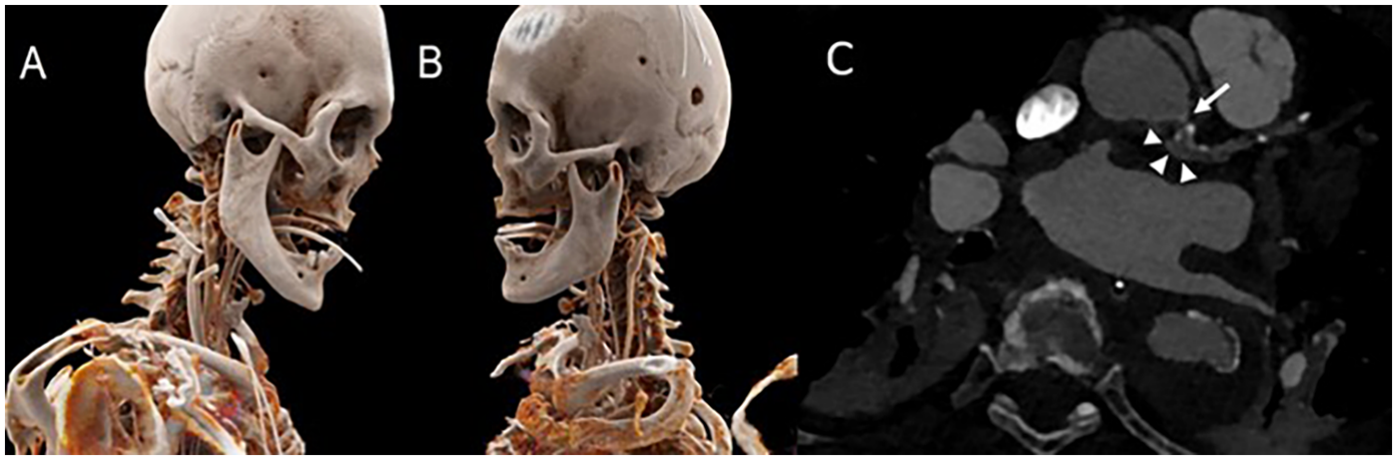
Postoperative CT-scan showing 3-D reconstructions of patient right (A) and left (B) A CT angiogram displaying a dissection membrane narrowing of the left main ostium (white arrow) and the false lumen (white arrowheads) (C).
